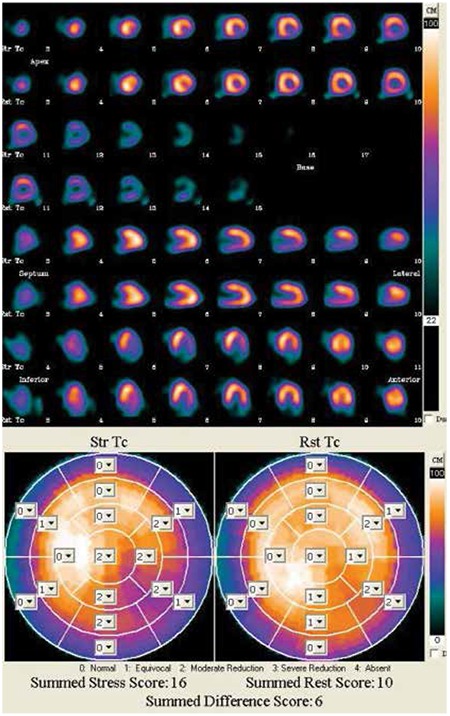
Stress and rest myocardial perfusion single-photon emission computed tomography images of a diabetic patient revealed reversible ischemia in the inferolateral wall
